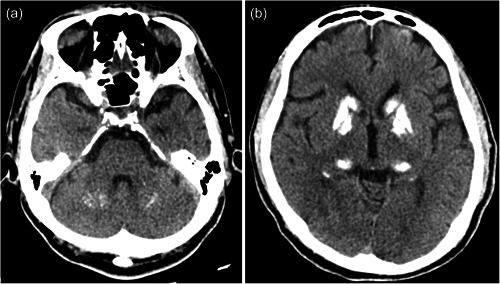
Evidence of bilateral calcification of the basal ganglia, thalamus, and dentate nucleus on brain computed tomography scan.
